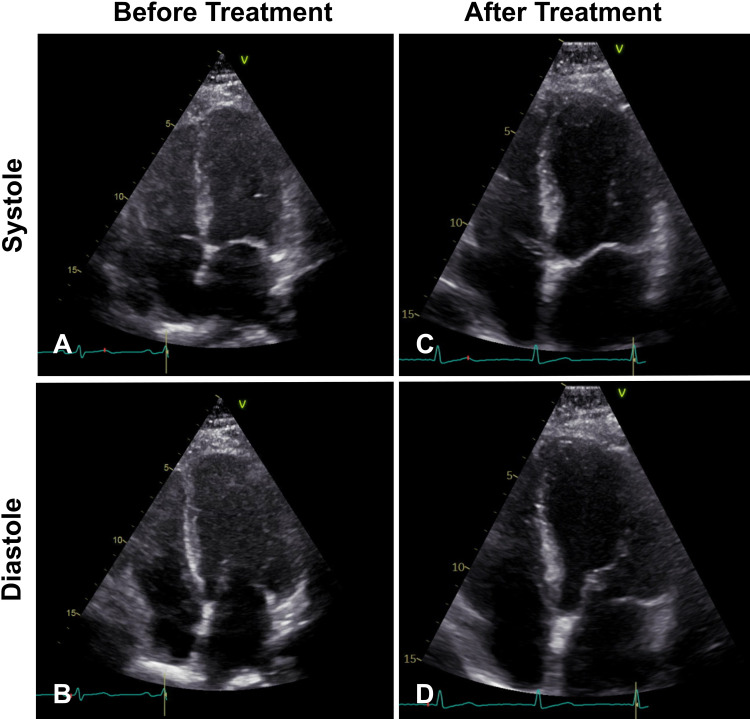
Echocardiograms before and after treatment Echocardiograms of patient’s heart before treatment (ejection fraction: 48%) (A, B) and after treatment (ejection fraction: 60%) (C, D) in systole and diastole, respectively
